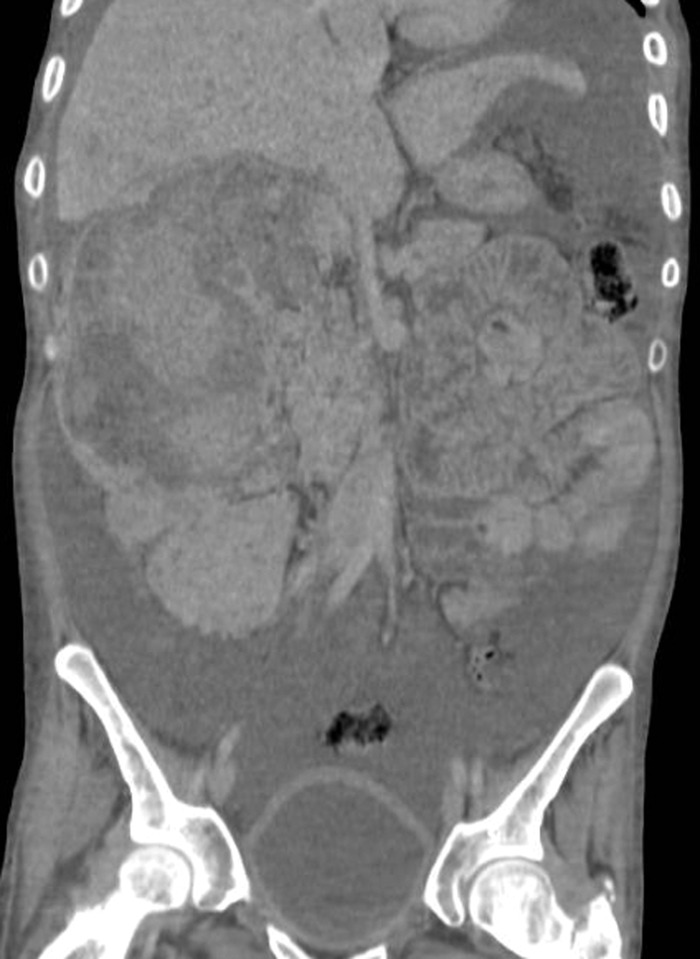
Computed tomography scan in coronal view shows a large right perinephric hematoma, left kidney with multiple angiomyolipomas, and abundant free fluid in the peritoneal cavity.
